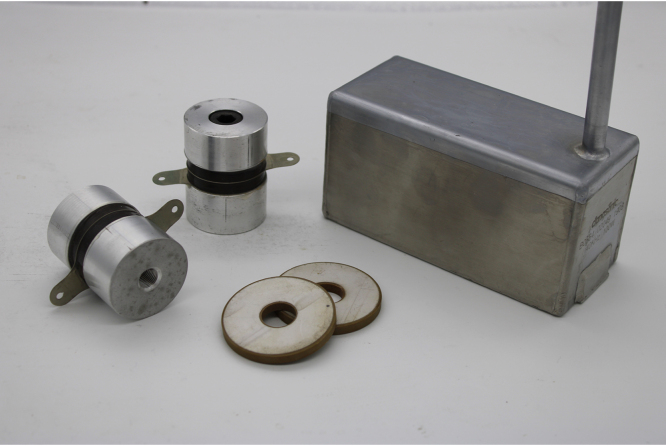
Langevin transducers and piezoelectric ceramics used to generate ultrasound.
